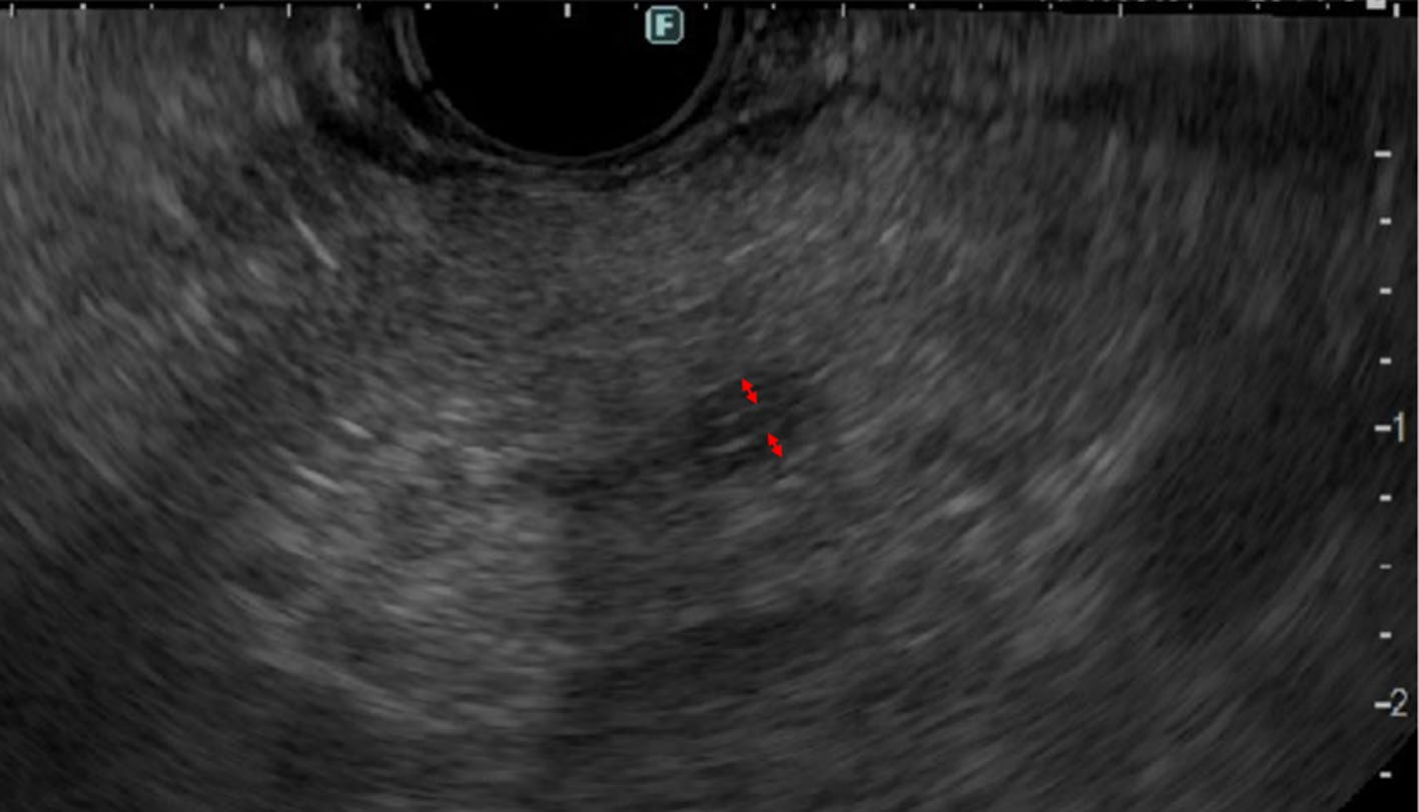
Endoscopic ultrasound showed no evidence of pancreatic parenchymal enlargement or mass lesion. However, the main pancreatic duct in the pancreatic body was sharply dilated to approximately 3 mm, with localized mural thickening. The site of ductal dilatation and mural thickening is indicated by double-headed arrows
